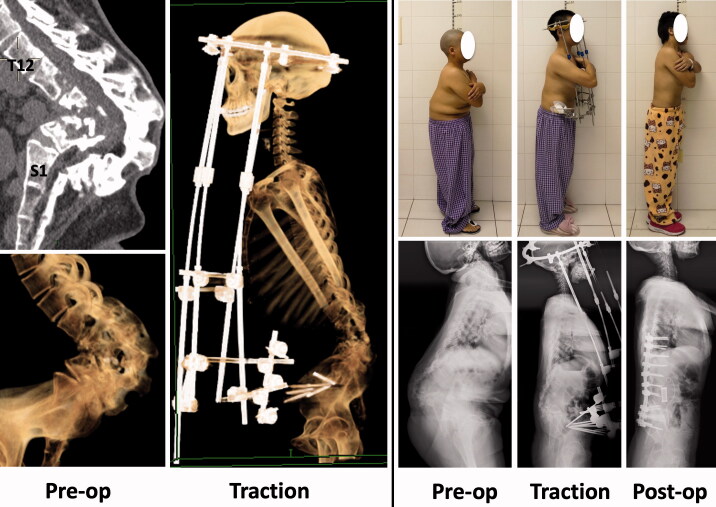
Case 2: CT revealed angular kyphosis in the lumbar region, almost complete absence of the vertebral bodies of L2 to L4, and complete fusion of the laminas of L1 to L5. Management consisted of HPT and anterior instrumentation and posterior fusion.
